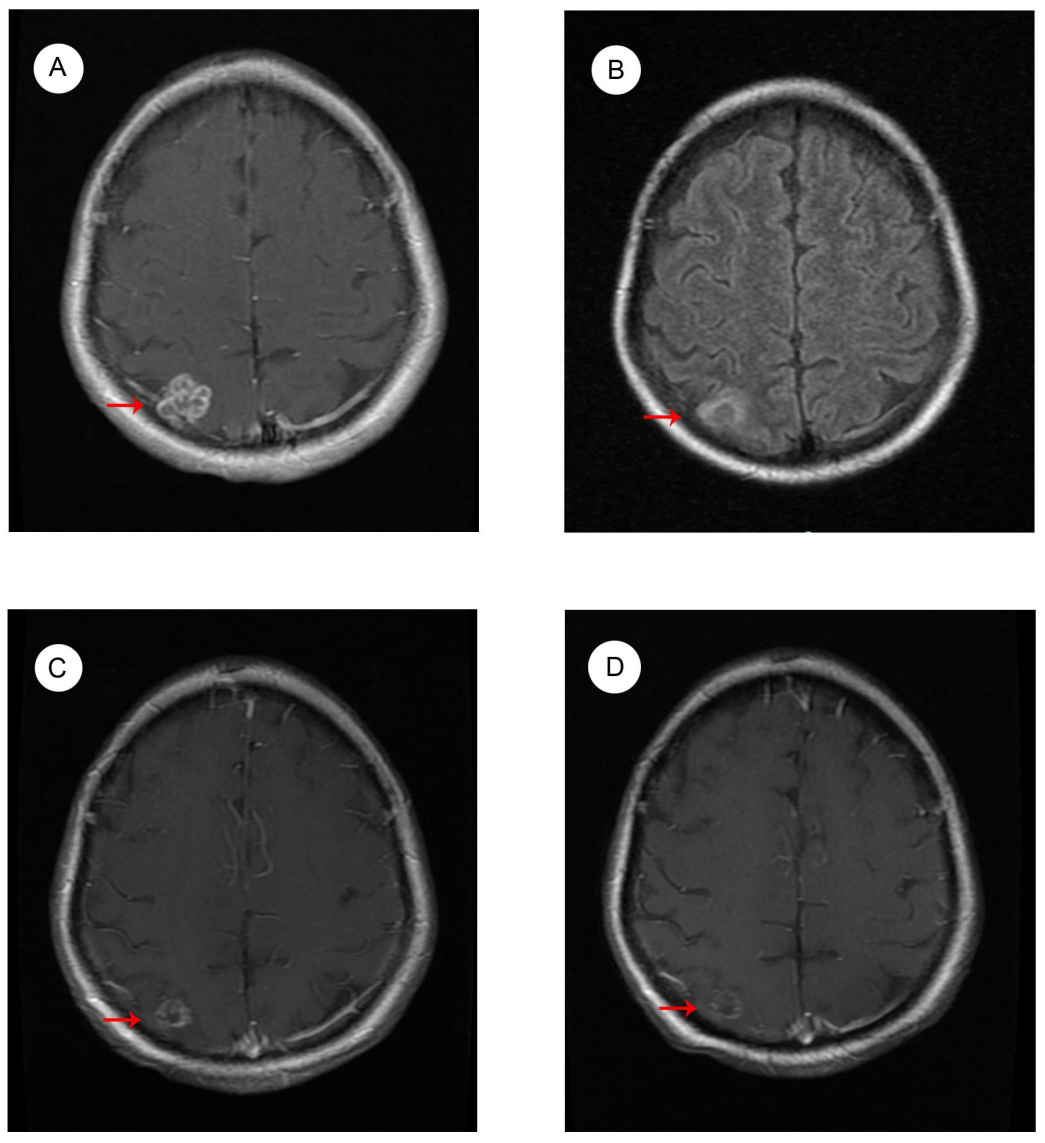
MRI images of brain metastases (A) before radiotherapy in December 2022; (B) after radiotherapy in January 2023; during Almonertinib + Pyrotinib treatment (C) in March 2023; and (D) in November 2023.
